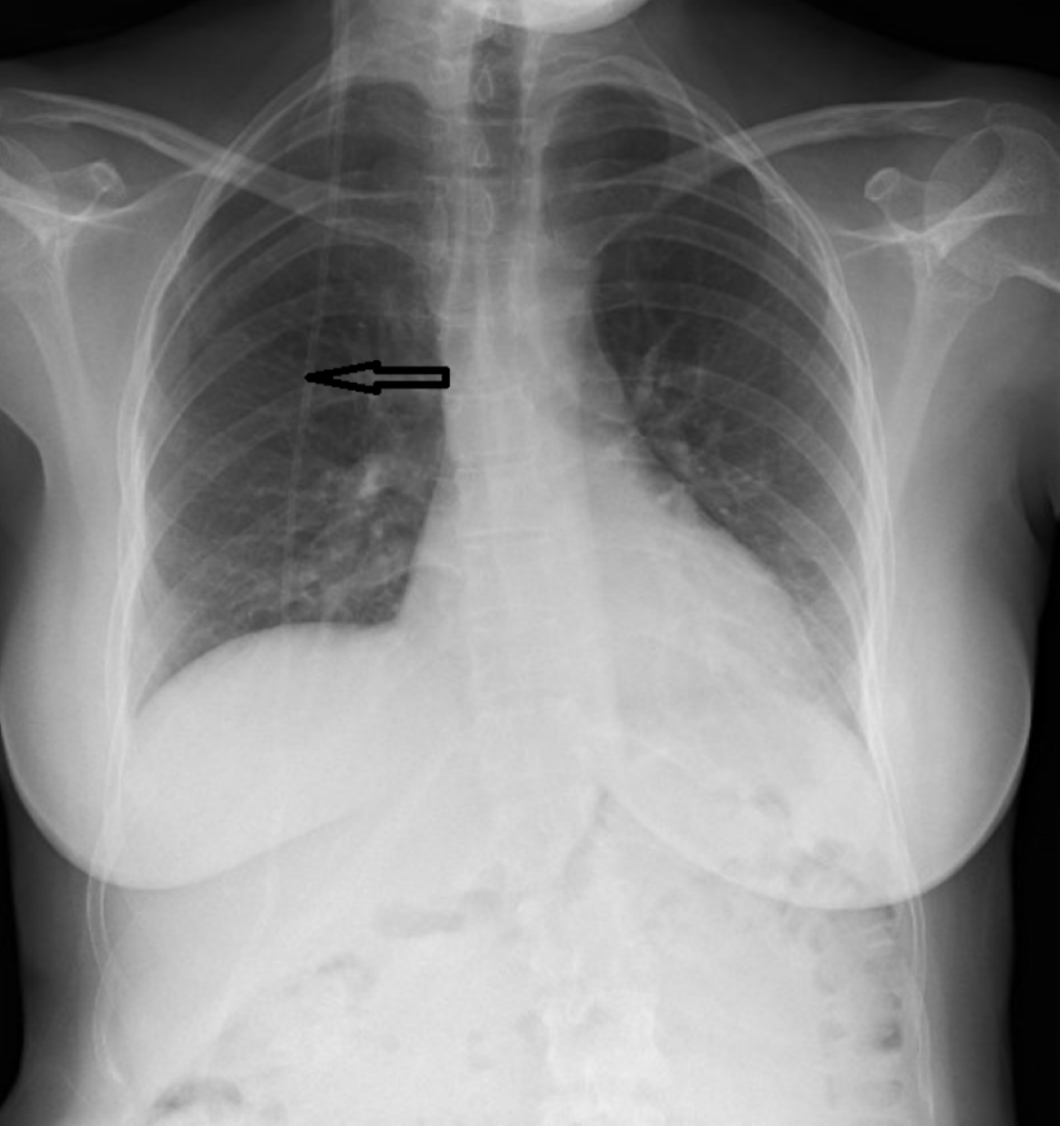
Chest X-ray Black arrow demonstrates ventriculoperitoneal shunt
